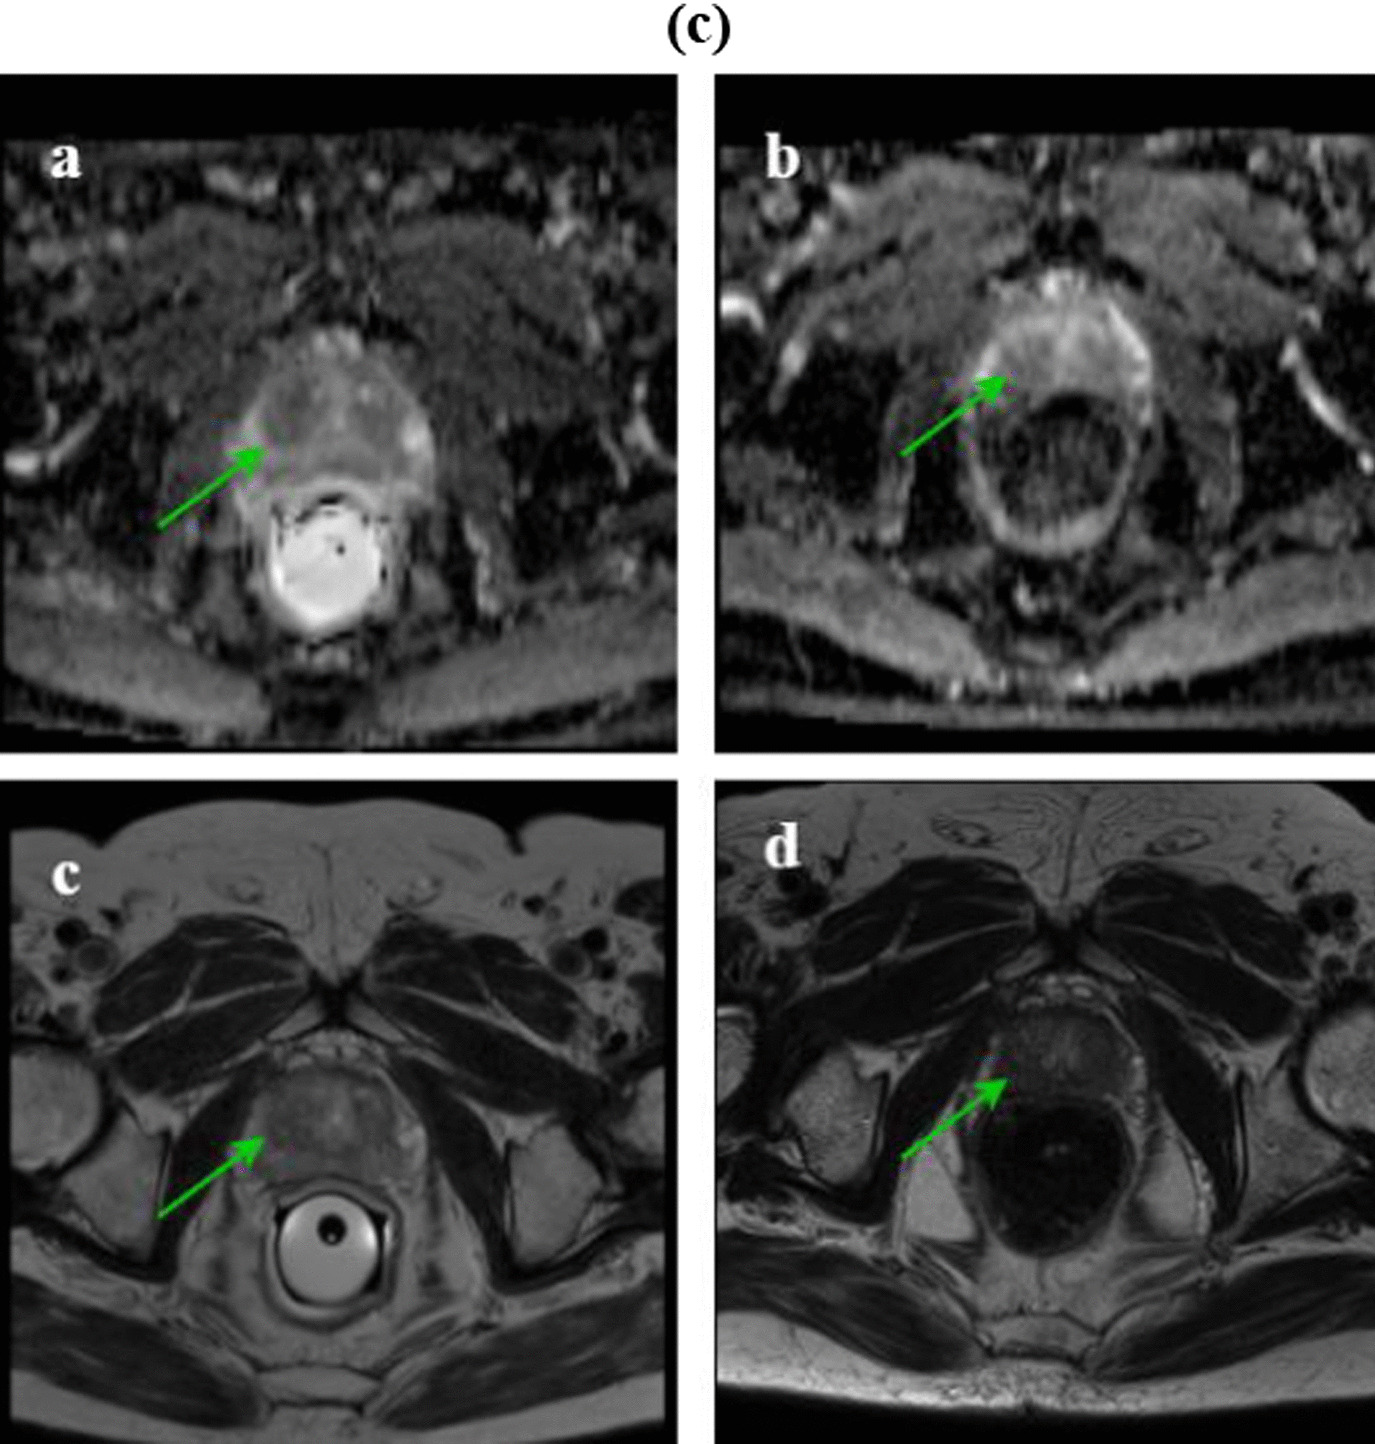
a The MR images of a 67-year-old man. Pre-radiotherapy, the axial T2W image shows a hypointense DIL in the right apex (c) and the DIL volume was 4.8 cm^3^ in the ADC image and the ADC value was 572 × 10^−6^ mm^2^/s (a). Post-radiotherapy, the axial T2W image shows the diffusely intermediate signal of the right apex (d) and the DIL volume was not detected in the ADC image, and the ADC value was 1210 × 10^−6^ mm^2^/s. These finding indicate a good response to the treatment (b). b The MR images of a 77-year-old man. Pre-radiotherapy, the axial T2W image shows a hypointense DIL in the right midgland (c) and the DIL volume was 4.6 cm^3^ in the ADC image and the ADC value was 820 × 10^−6^ mm^2^/s (a). Post-radiotherapy, the axial T2W image shows a diffusely intermediate signal of the right midgland (d) and the DIL volume was 0.38 cm^3^ in ADC image, and the ADC value was 920 × 10^−6^ mm^2^/s. These findings indicate a poor response to the treatment (b). c The MR images of a 64-year-old man. Pre-radiotherapy, the axial T2W image shows a hypointense DIL in the left peripheral zone (c) and the DIL volume was 1.2 cm^3^ in the ADC image and the ADC value was 651 × 10^−6^ mm^2^/s (a). Post-radiotherapy, the axial T2W image shows the diffusely intermediate signal of the left midgland (d). Post-radiotherapy, the DIL volume was 0.72 cm^3^ in ADC image and the ADC value was 736 × 10^−6^ mm^2^/s. These findings indicate a poor response to the treatment (b)
